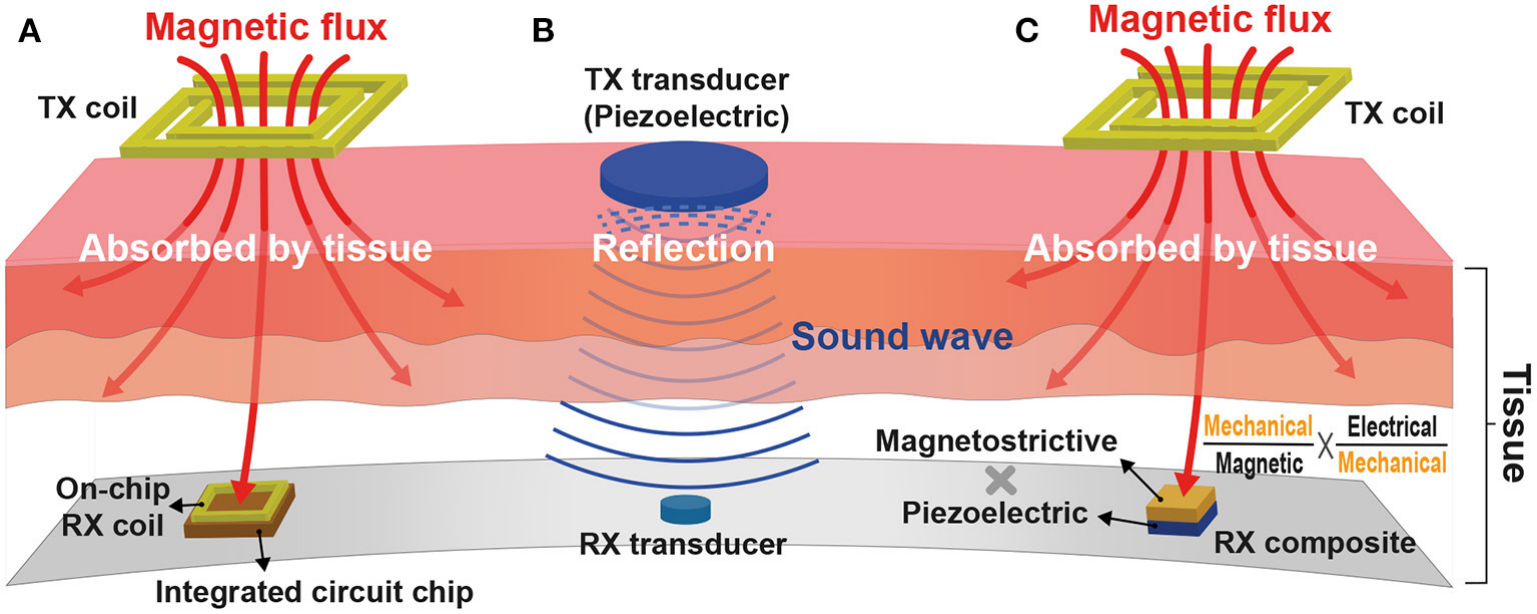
Wireless power transfer (WPT) modalities for a miniaturized implant: (A) Inductive WPT using an on-chip power receiving coil; (B) Ultrasonic WPT having a short wavelength; and (C) Magnetoelectric WPT integrating both inductive and ultrasound WPTs.
